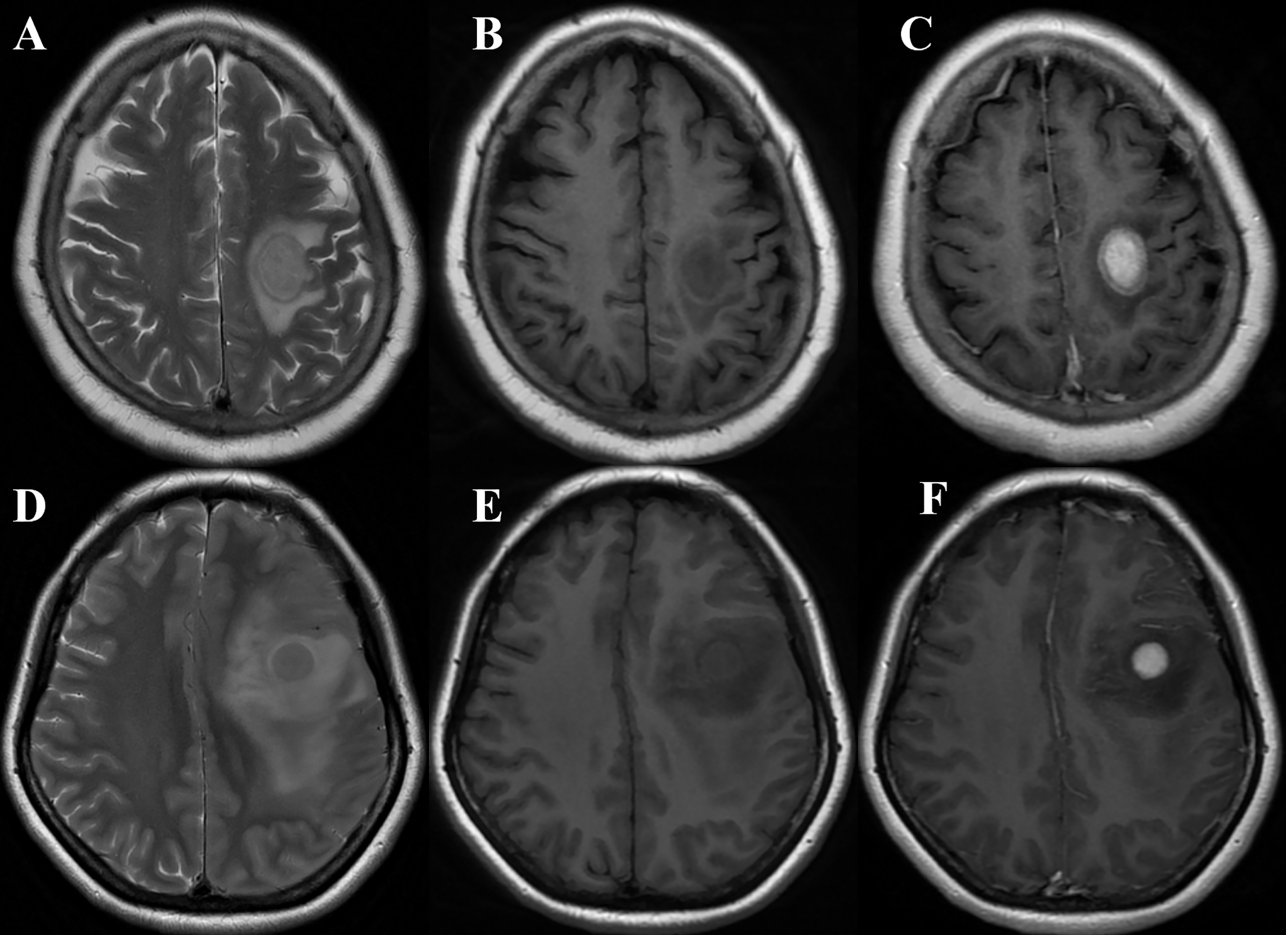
(A–C) A 68-year-old woman with primary central nervous system lymphoma (PCNSL) presented with left hemiparesis for 1 month. MRI showed a left frontal lobe lesion with iso- to slight hyperintensity on T_2_WI (A), slight hypointensity on T_1_WI (B), and marked homogeneous enhancement on T_1_CE imaging (C) (take gray matter for reference). The quantitative parameters showed that rT1, rT2, T2/T1, and rT1CE were 0.65, 1.20, 1.82, and 1.87, respectively. The case was correctly diagnosed as PCNSL by models 1, 2, 4, and 5 and radiologist B while wrongly classified as glioblastoma (GBM) by radiologist A. (D–F) A 43-year-old woman with GBM presented with seizure. MRI showed a left frontal lobe lesion with isointensity on T_2_WI (D), slight hypointensity on T_1_WI (E), and marked homogeneous enhancement on T_1_CE imaging (F) (take gray matter for reference). The quantitative parameters showed that rT_1_, rT_2_, T_2_/T_1_, and rT1CE were 0.66, 1.46, 2.25 and 2.11, respectively. The case was correctly diagnosed as GBM by models 1, 2, 4, 5, and 6 and radiologist B while wrongly classified as PCNSL by radiologist A.
